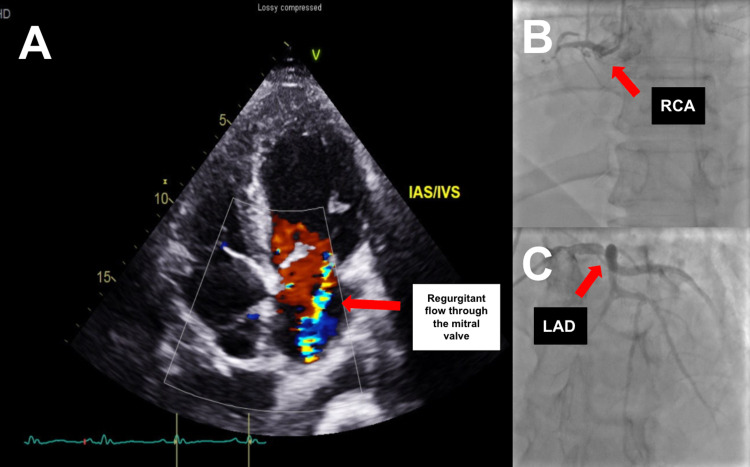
(A) Transesophageal echocardiogram prior to coronary artery bypass surgery and mitral valve replacement demonstrating regurgitant flow through the mitral valve shown by the red arrow. (B-C) Cardiac catheterization demonstrating severe stenosis in the proximal left anterior descending (LAD) artery as well as a chronic total occlusion in the proximal right coronary artery (RCA) and obtuse marginal arteries shown by the red arrows.
